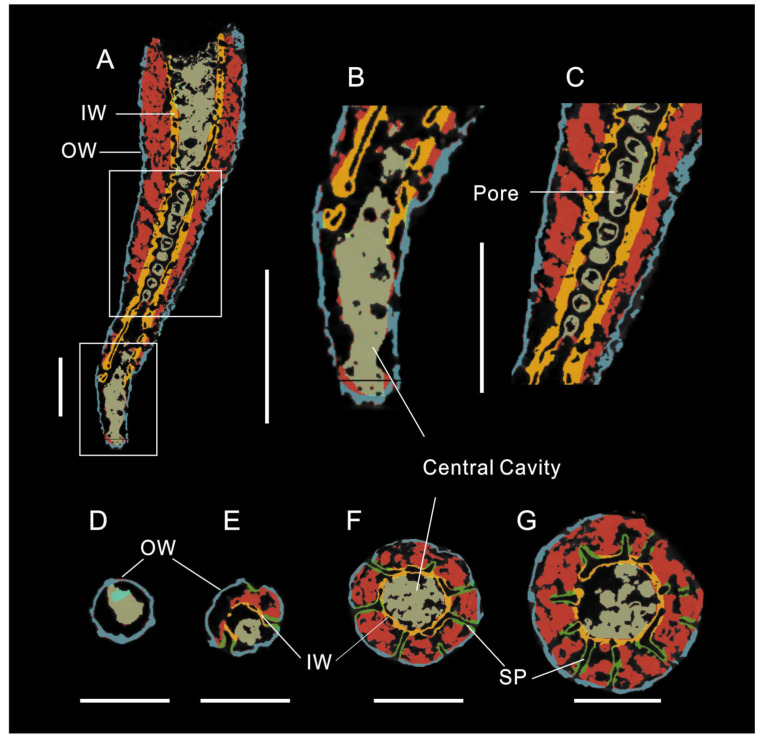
X-ray micro-computer tomography images of Ajacicyathida. ELI-AC-040. (A) Anatomical structure of the cup showing two walls with pores, intervallum, and central cavity. (B) Enlarged view of lower boxed area in (A) showing the lower part of the cup with only outer wall. (C) Enlarged view of upper boxed area in (A) showing the inner wall pores. (D–G) The transverse view of cup showing the septa perpendicular to the two walls at different stages. (D) Only outer wall at initial stage. (E) Two walls and three septa. (F) Two walls and seven septa. (G) Showing the two walls and ten septa. OW: outer wall; IW: inner wall; SP: septa. All scale bars = 500 μm.
